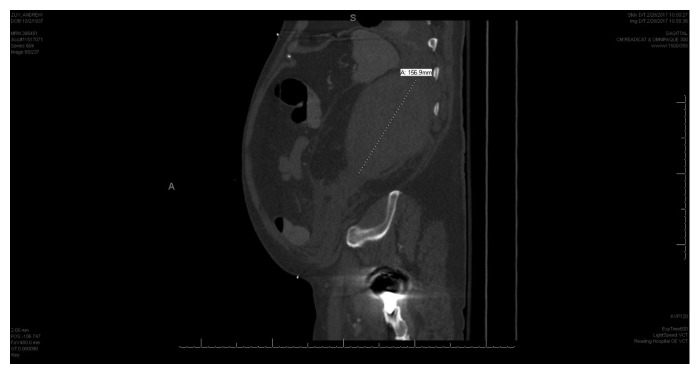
CT scan of the abdomen with retroperitoneal hematoma.
